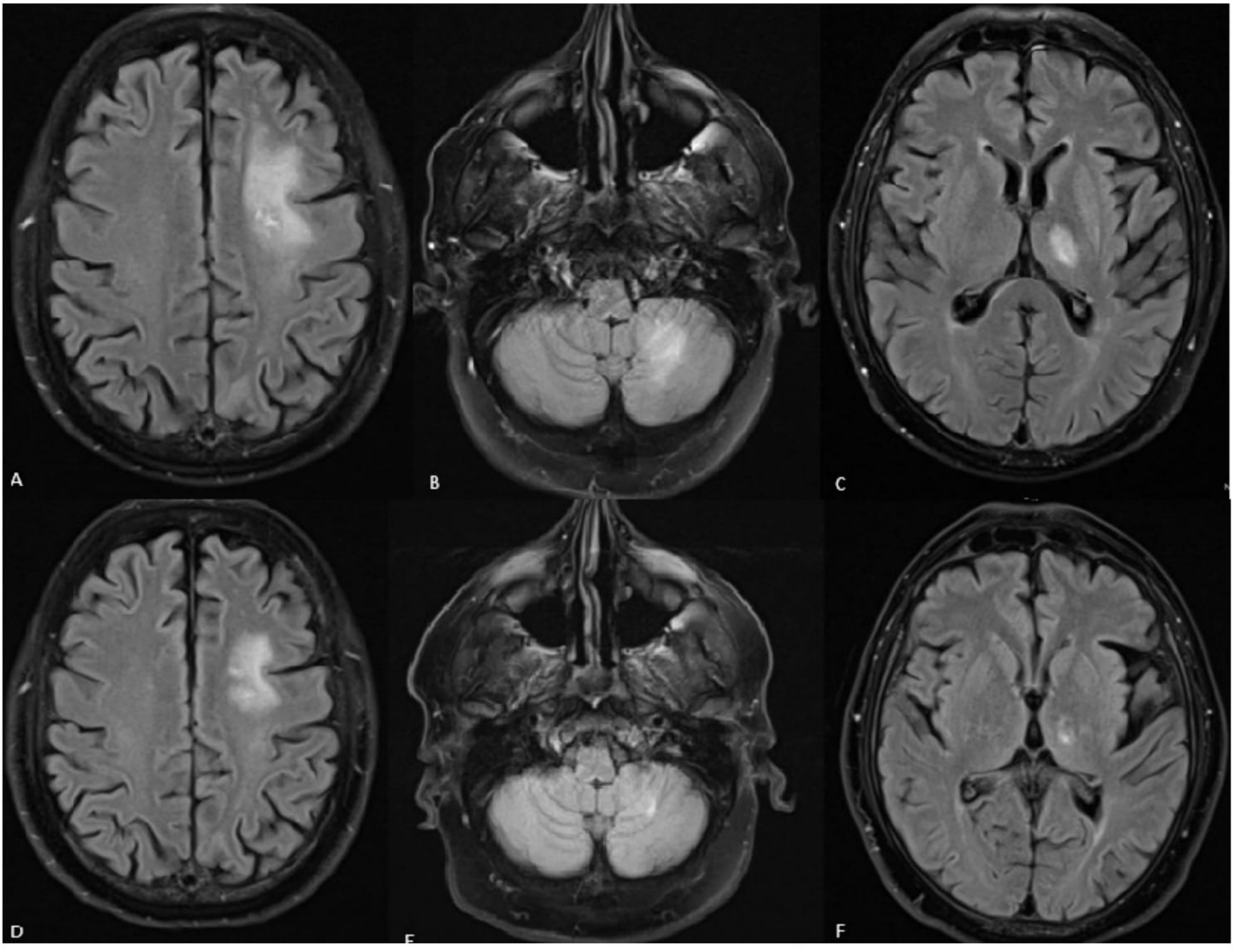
Brain MRI findings in *case n.1*, FLAIR sequencing. A-B-C: baseline sequences showing hyperintensity in the left fronto-parietal area, left cerebellar region and left thalamus respectively. D-E-F: sequences obtained after 2 weeks of steroid treatment revealed significant improvement and a reduction in lesion size
